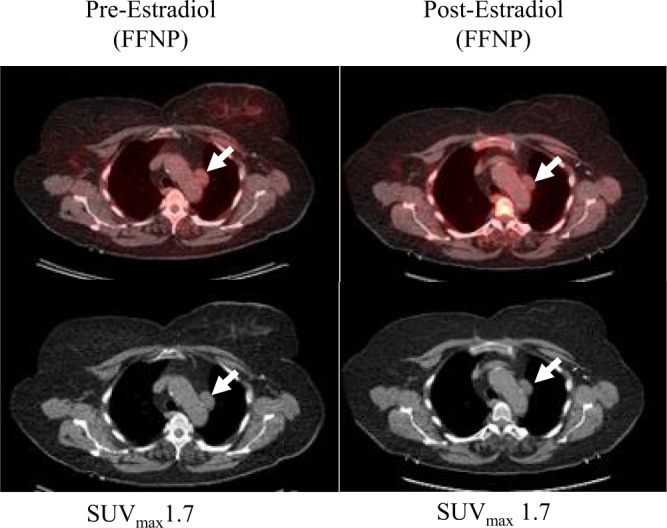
Nonresponder. A woman with ER+/PR−/HER2− invasive ductal carcinoma post neoadjuvant ET and breast conserving surgery, who developed metastatic disease to left prevascular and aortopulmonary lymph nodes. She was treated with an aromatase inhibitor after the estradiol challenge test but developed progressive disease. Selected fused transaxial FFNP-PET/CT (top) and CT (bottom) images at baseline show minimal FFNP uptake in the prevascular lymph node metastasis (arrows). One day after estradiol, tumor FFNP uptake measured as the maximum standardized uptake value (SUV_max_) (arrows) is unchanged.
